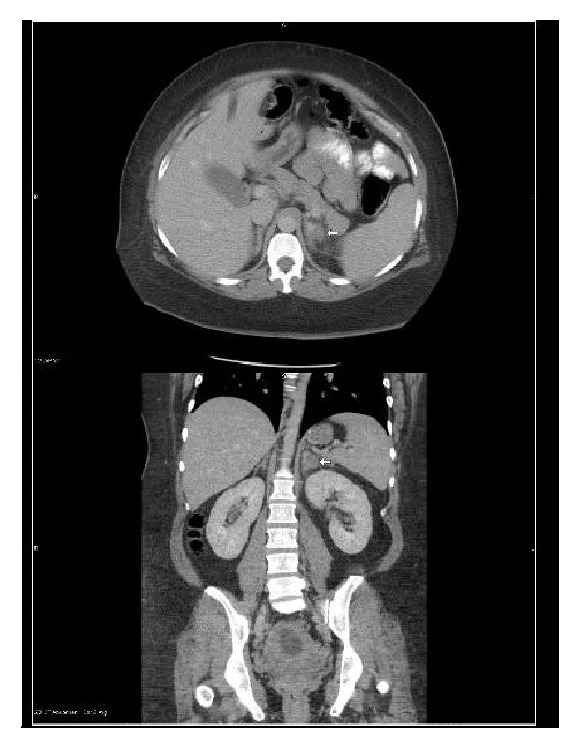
CT scan findings with arrow pointing at left adrenal hemorrhage.
